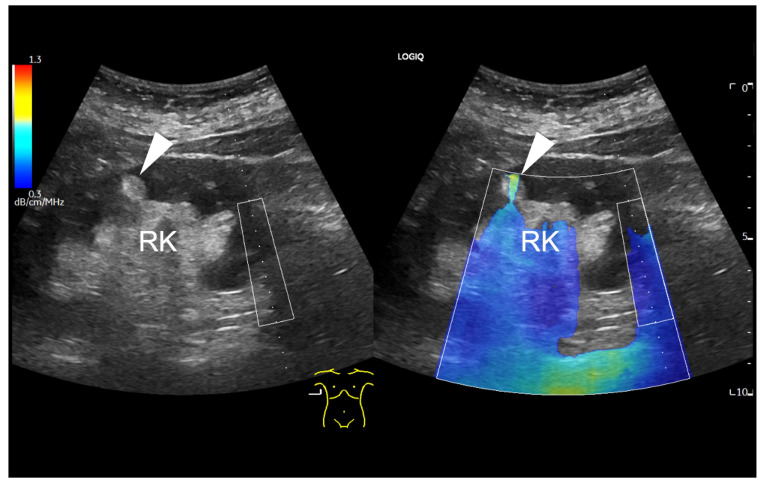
B-mode ultrasound of the right kidney (RK), displaying a focal hyperechogenic lesion (arrowhead) in the cortex, which is barely included in the colour coded attenuation map. The ROI box on the right side of the map can be only moved horizontally due to methodological reasons. Due to the superficial localization of the small renal lesion, the attenuation colour map is only partial applicable (arrowhead).
